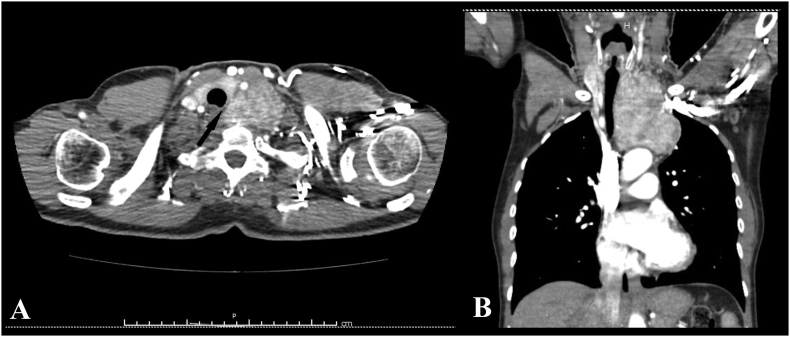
A, B. CT-scan showing a 90 × 80 × 50 mm mass, extending from the cervico-thoracic junction to the aortic arch. The lesion appears as highly vascularized and heterogeneous, contiguous but clearly separated from the cervical thyroid gland (arrow), which shows no abnormalities. Trachea, esophagus and left carotid and subclavian arteries are compressed and displaced.
